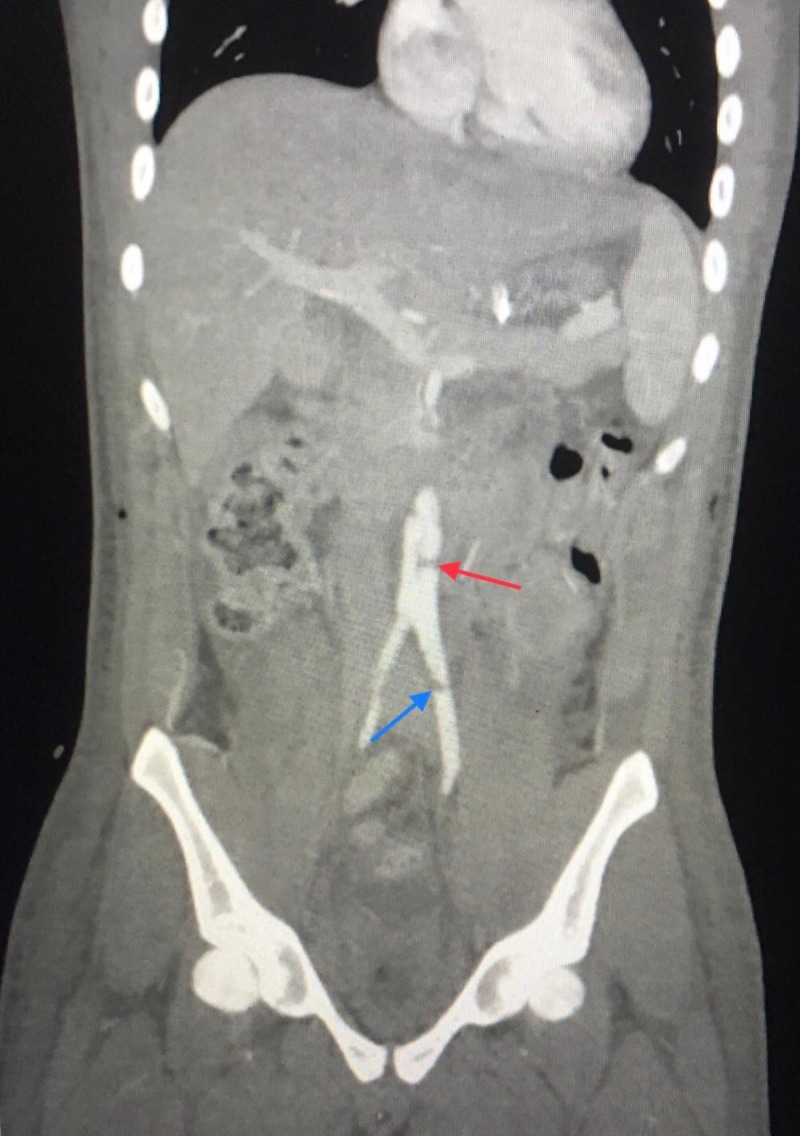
Computed tomography angiography of abdomen and pelvis with run off Aortic dissection and clot (red arrow) Left common iliac clot (blue arrow)
